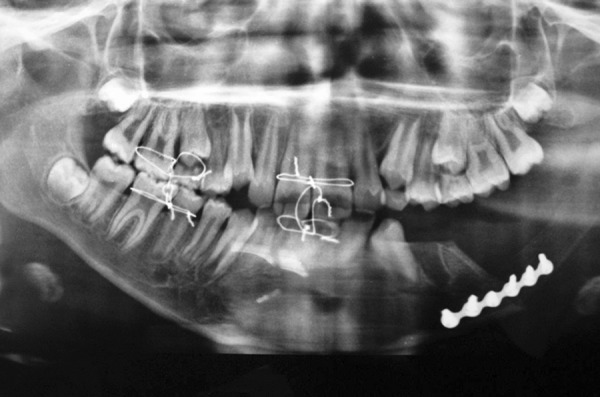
Radiograph to ensure the stability of the graft
